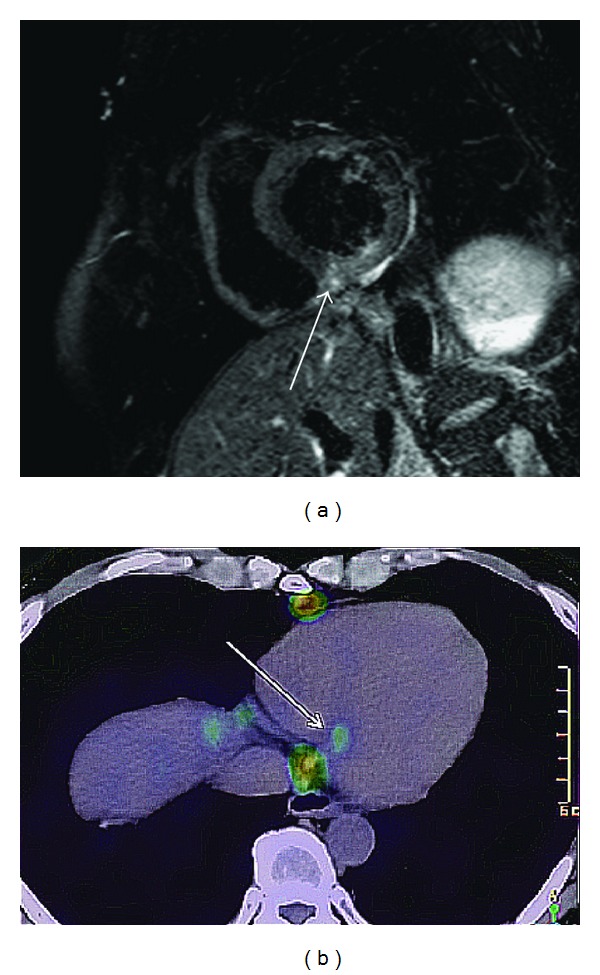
41-year-old male with cardiac sarcoidosis presenting with premature ventricular contraction. Short-axis T2-weighted MRI (a) shows a small myocardial edema at the inferior septal myocardium (arrow). ^18^FDG-PET (b) shows the focal inflammation (arrow), consistent with the edema on MRI.
